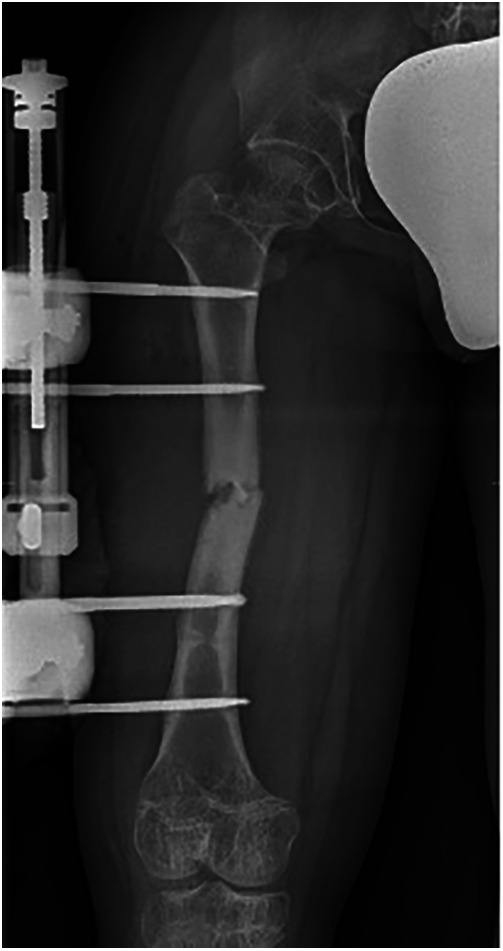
Postoperative radiograph after placement of a uniaxial external fixator following diaphyseal fracture of the right femur.
